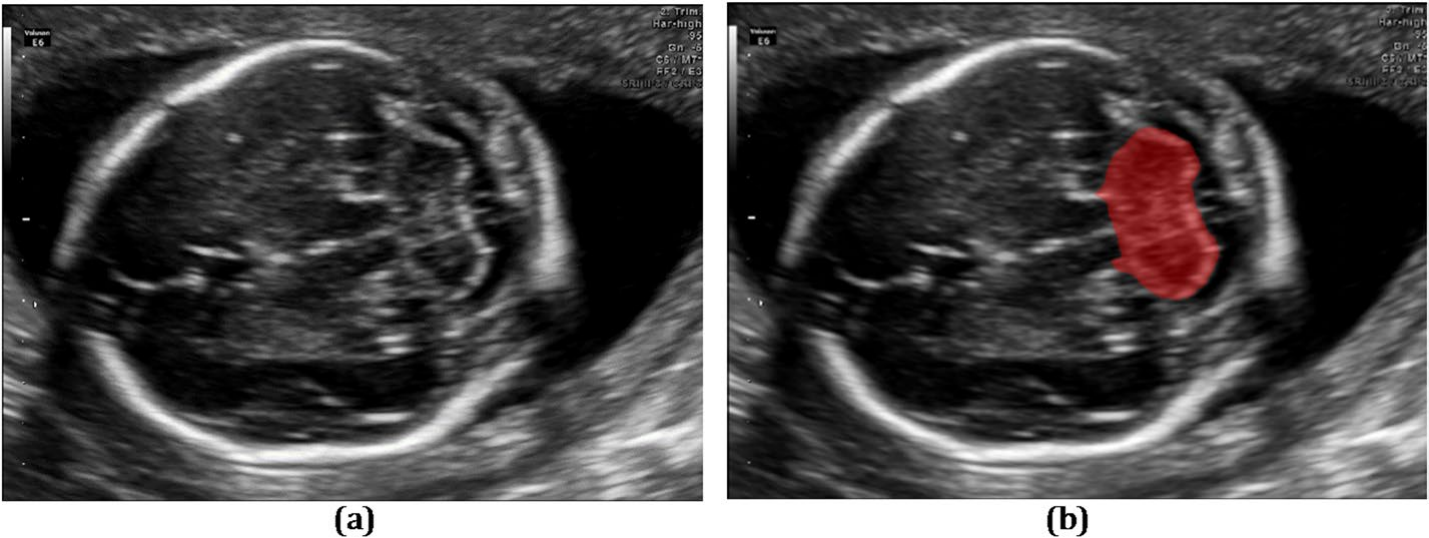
The cerebellar region in an ultrasound image. (a) Original image. (b) Highlighted cerebellar area.
